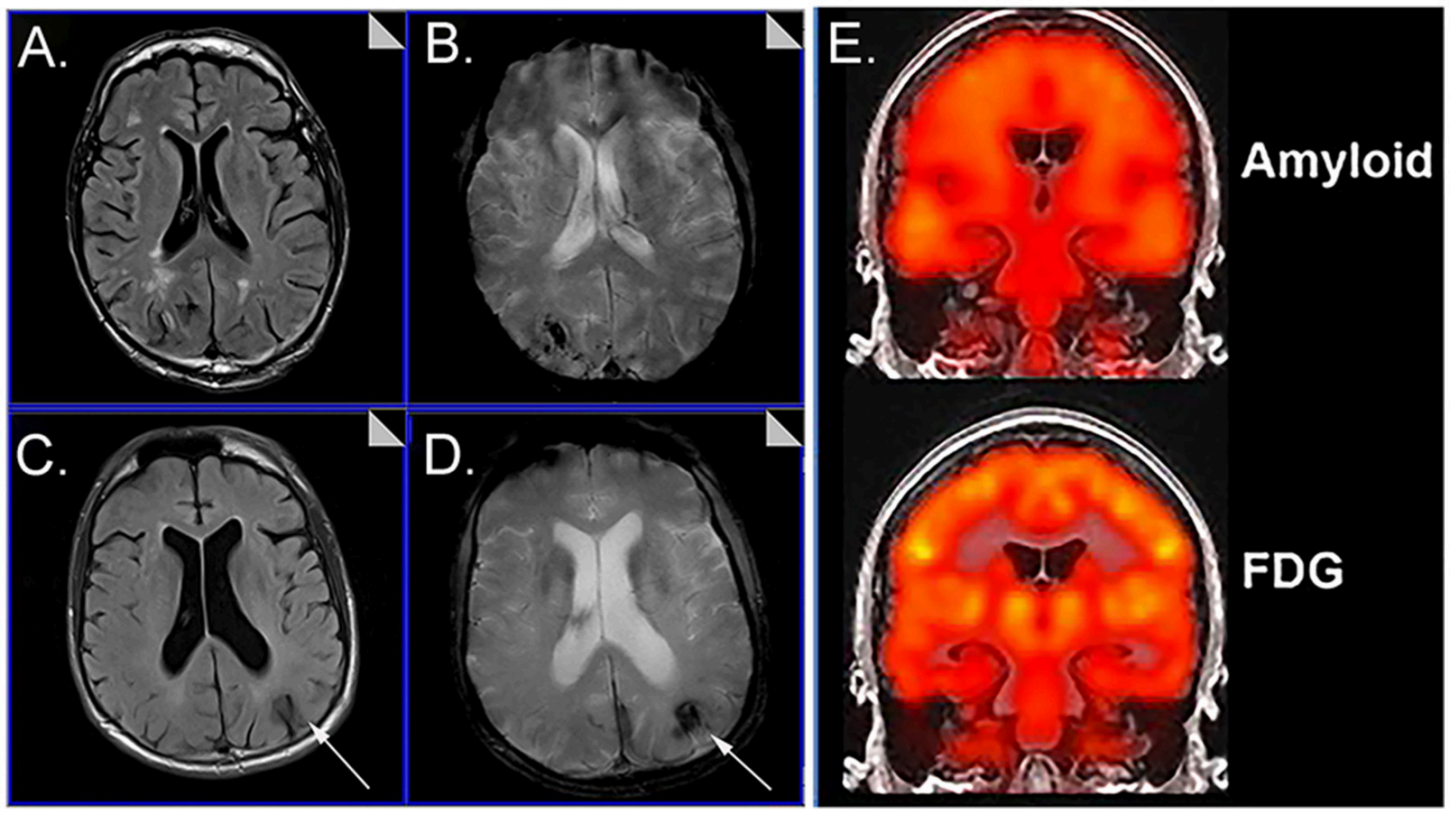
Representative examples of neuroimaging protocols acquired in Down syndrome. Panels (A–D) show MR imaging in a 57 year old male and 59 year old male imaged with T2^*^
